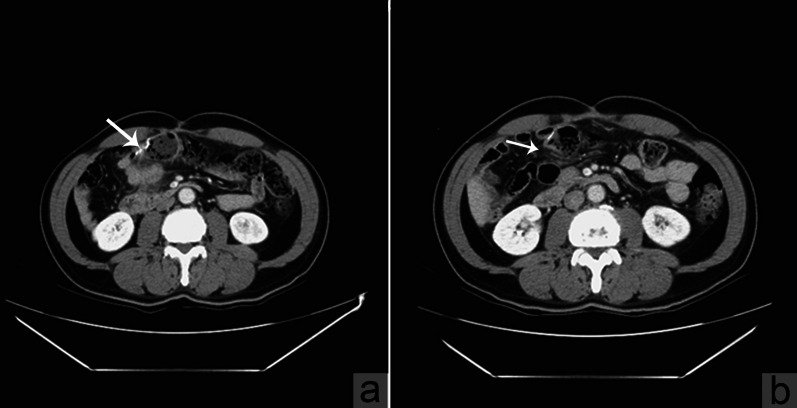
Contrast-enhanced abdominal CT study showed a a moderate contrast enhancement with apparent desmoplastic reaction near previous surgical sutures (white arrow); and b complete lesion clearance three months after surgery (white arrow)
